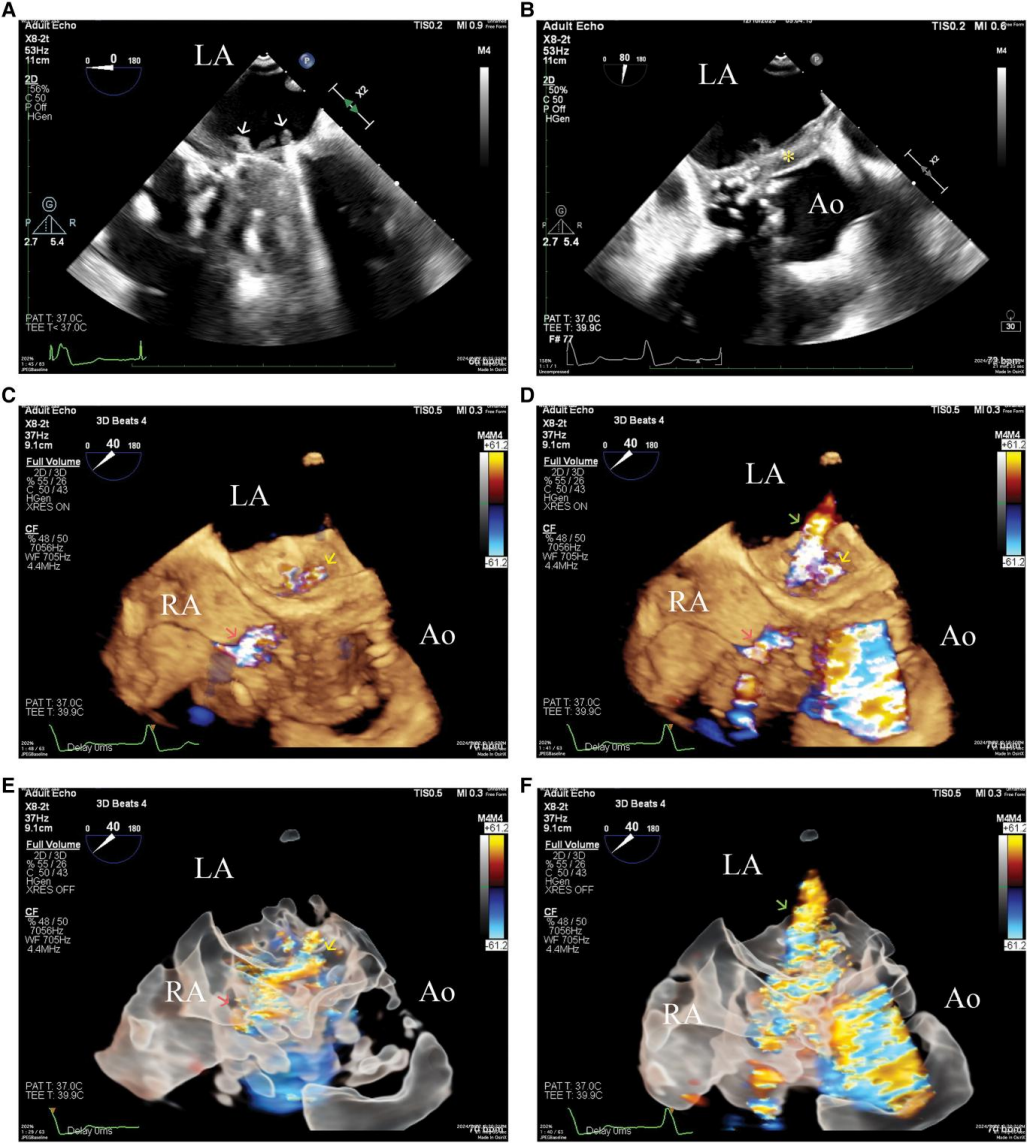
(*A*) 2D transoesophageal echocardiography showing vegetations (arrows) involving the prosthetic mitral valve in the four-chamber view. (*B*) 2D transoesophageal echocardiography showing echo-dense thickening (asterisk) of the aortic peri-valvular tissue. (*C*) 3D transoesophageal echocardiography with colour Doppler in the *diastolic phase* shows blood flow shunting of the aorto-cavitary fistulization into the left atrium (yellow arrow) and right atrium (red arrow). (*D*) 3D transoesophageal echocardiography with colour Doppler in the *systolic phase* shows a paravalvular leakage jet of the prosthetic mitral valve (green arrow), in addition to the blood flow shunting of the aorto-cavitary fistulization into the left atrium (yellow arrow) and right atrium (red arrow). (*E*) 3D glass view with colour Doppler in the *diastolic phase* delineates the continuity of the blood flow shunting into the left atrium (yellow arrow) and right atrium (red arrow). (*F*) 3D glass view with colour Doppler in the *systolic phase* shows a paravalvular leakage jet of the prosthetic mitral valve (green arrow), in addition to the aorto-cavitary fistulization.
